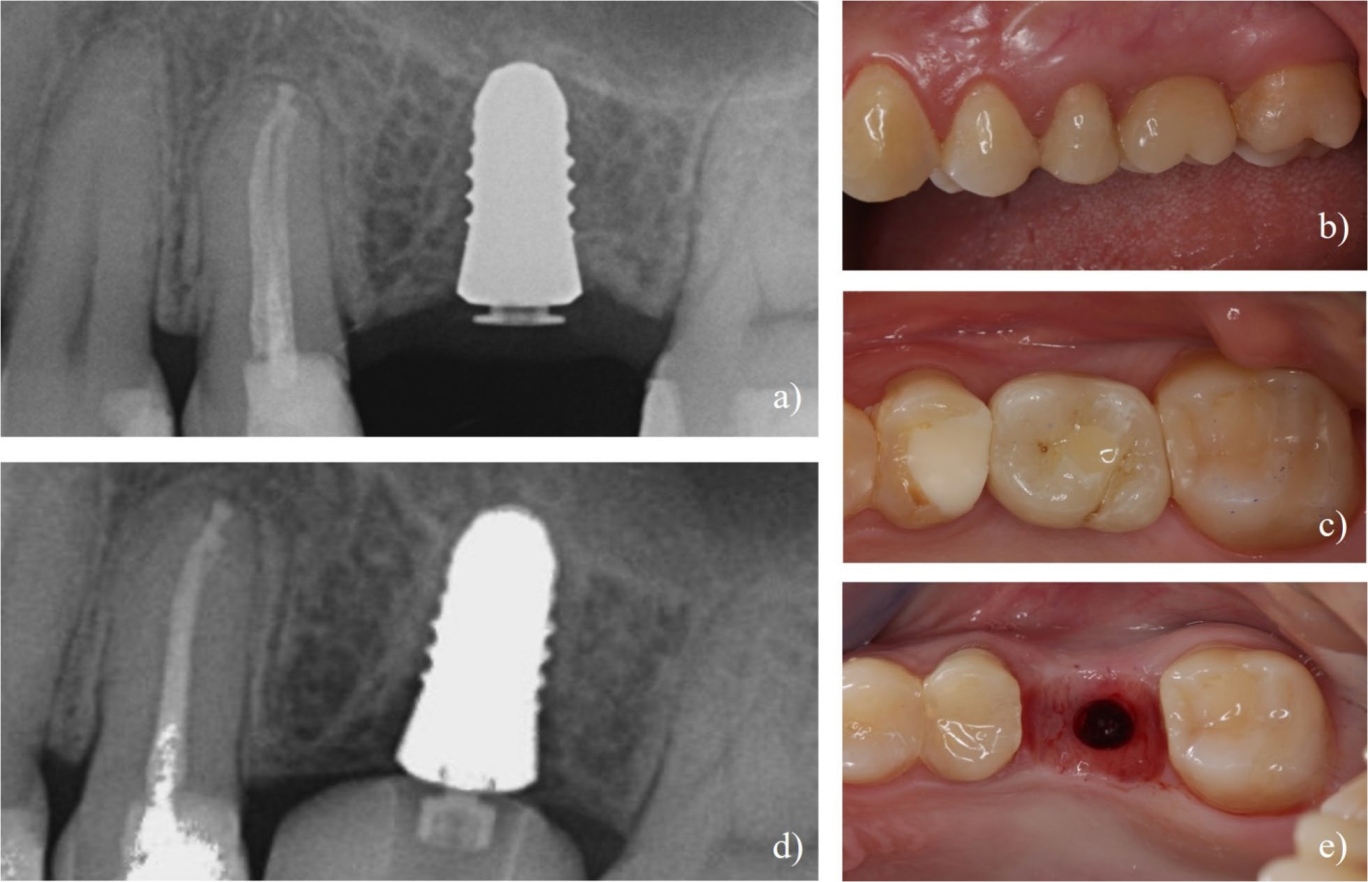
Case of implant loss due to implant mobility. a Initial radiograph showing dental implant with definitive screw retained crown and translucent PEKK abutment. b, c Clinical view of the implant-supported crown in situ at time of definitive prosthesis placement. d Follow-up radiograph revealing peri-implant radiolucency, indicative of implant failure due to loss of osseointegration. e Clinical appearance after implant removal, showing extraction socket
